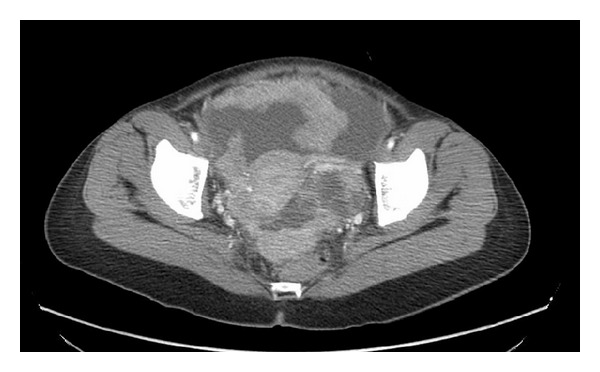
CT scan. Pelvic mass with ascites, peritoneal deposits, and enlargement of the greater omentum.
